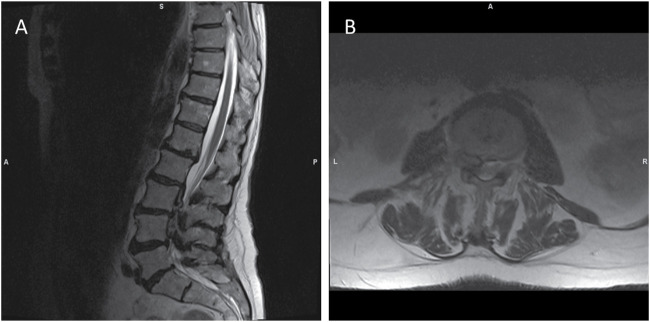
Preoperative Non-Contrast T2-Weighted MRI Sequence. (A) The Sagittal Image Reveals Multisegmental Disc Bulging and Intervertebral Disc Herniations at the Endplates of L2 and L3, as Well as at L3/4. Degenerative Changes are Observed at the L1/L2 and L2/L3 Endplates, With Activated Osteochondrosis Noted at L1/L2. Severe Spinal Canal Stenosis is Evident at L3-L5, with the Most Pronounced Narrowing Along the Posterior Edge of L4 and at L4/5. (B) Axial Imaging at L4 Demonstrates an Extradural Mass Occupying the Anterior Spinal Canal, Resulting in Significant Radicular Compression and Severe Canal Stenosis. The Intraspinal Mass Appears Hyperintense and is Surrounded by a Hypointense Rim.
